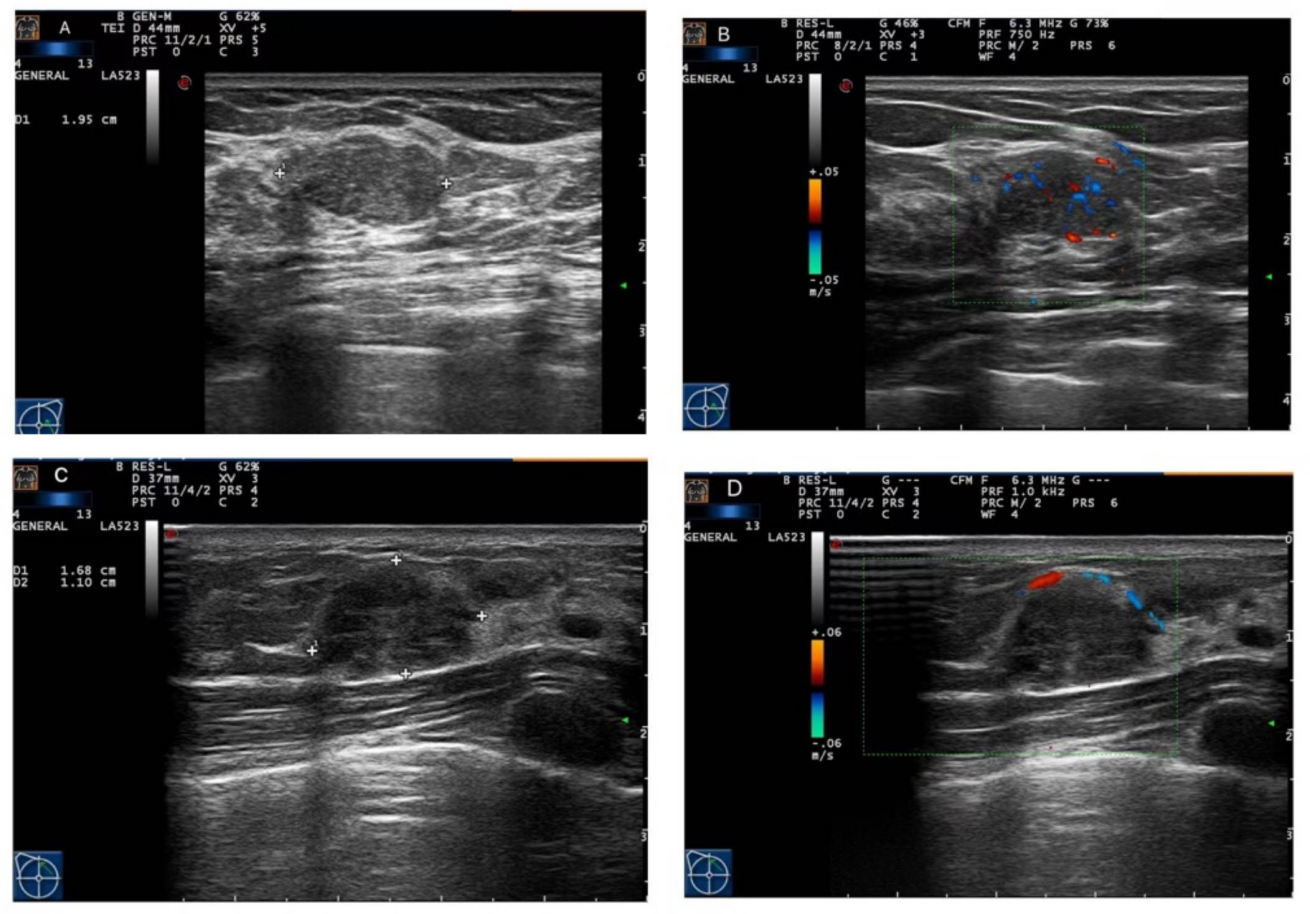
Ultrasound imaging of fibroadenoma (FA) and pure mucinous carcinoma (P-MC). (A, B) Images from a 46-year-old female patient. Ultrasound imaging showed that there was a hypoechoic nodule with a regular shape and circumscribed edge and rich blood flow in the left breast, which was confirmed as fibroadenoma by pathology. (C, D) Images from a 33-year-old female patient. Ultrasound imaging showed that there was a hypoechoic nodule with a regular shape and circumscribed edge and poor blood flow in the right breast, which was confirmed as pure mucinous carcinoma by pathology.
